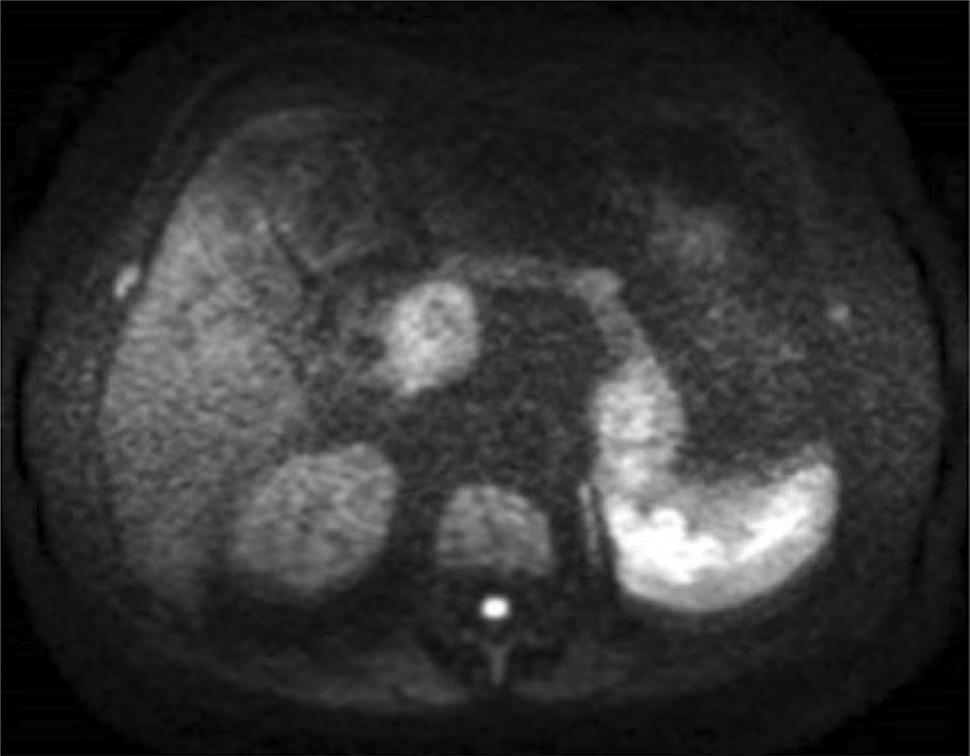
Diffusion-weighted magnetic resonance imaging (b-factor = 1000 s/mm2) showed multiple tumors in the pancreas with a high signal intensity. Apparent diffusion coefficient map revealed pancreatic tumors with low intensity
